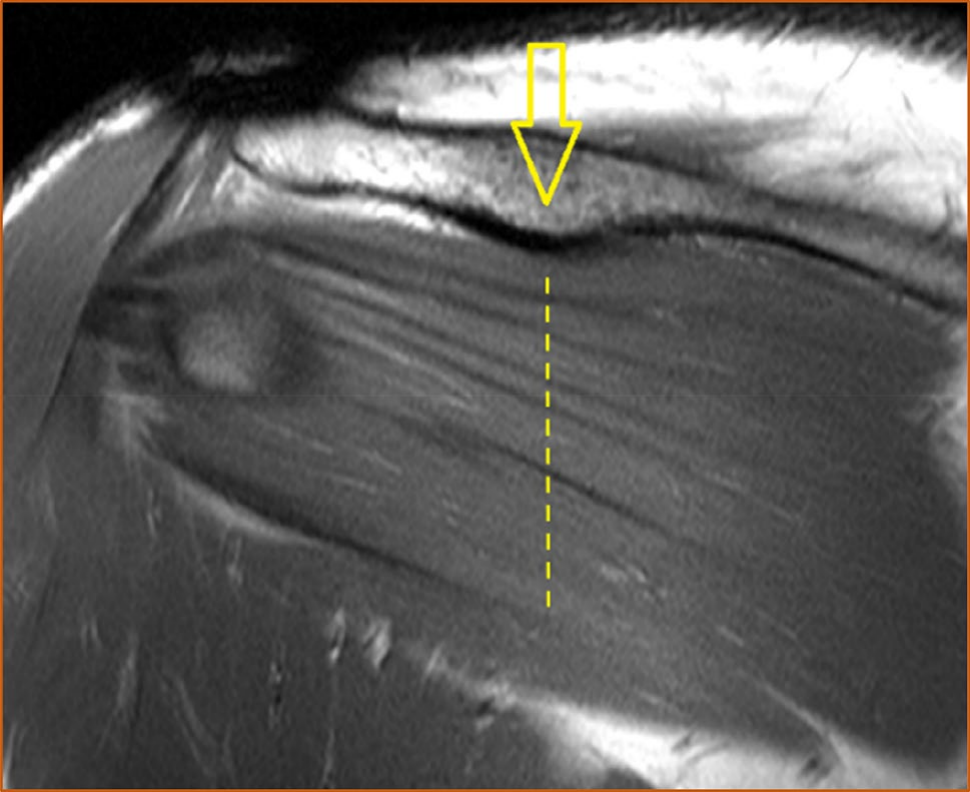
The intramuscular tendons of the infraspinatus muscle were counted in a vertical line (dashed above the arrow) midway between the glenohumeral joint space (left-most line) and the insertion of the muscle (right-most line) in the coronal view. The line above the arrow was labeled "the lateral measuring point". T1-weighted image, turbo spin echo. Coronal section
